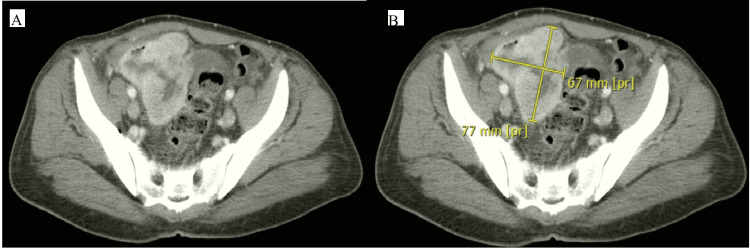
CT scan of the abdomen and pelvis with an intra-abdominal mass. The left image is without a marker, and the right image with a mark points to the mass, which is a pelvic intra-abdominal mass that measures about +/- 7.5x8x9 cm in maximal diameter.
